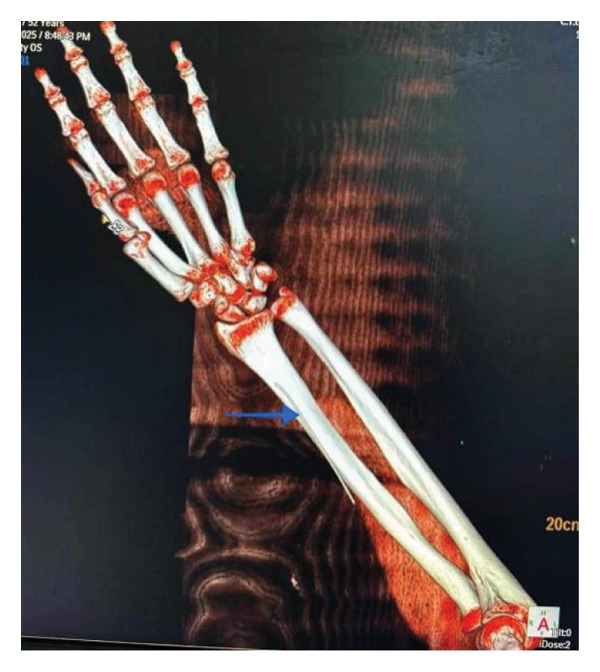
CTA of the left forearm showing retained catheter fragment (blue arrow) ∼3 cm proximal to radial styloid process.
